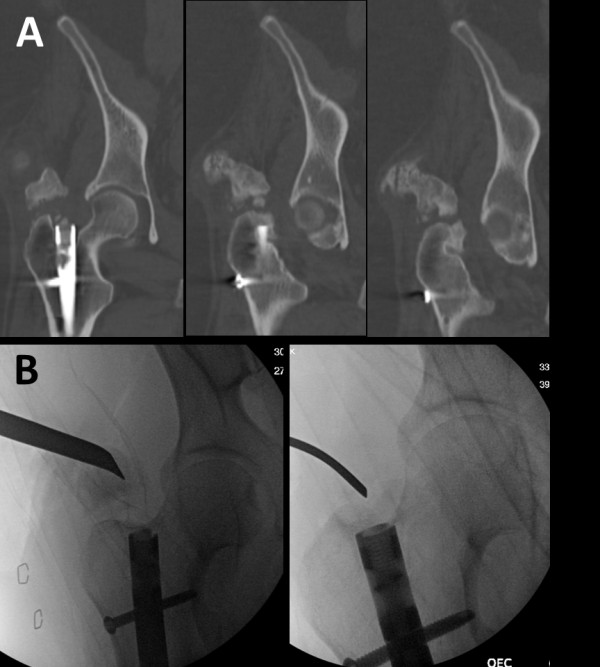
Preoperative computed tomography (CT) scan (A) and intraoperative fluoroscopy images during the surgical resection procedure (B).
